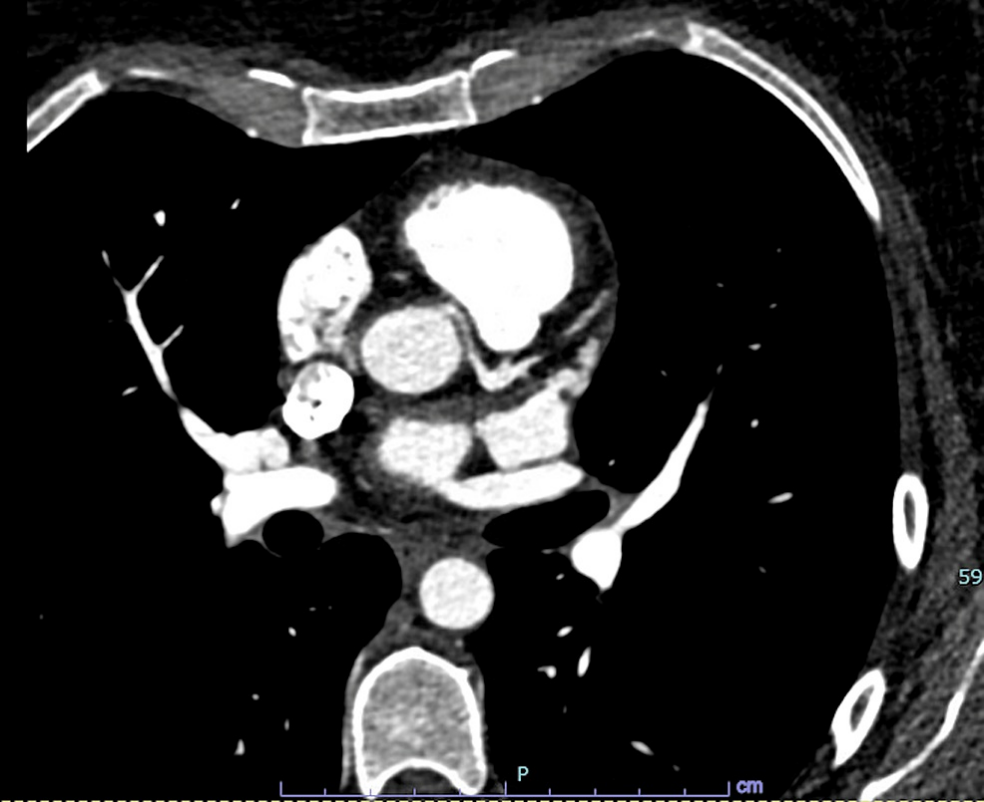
CT Angiogram Demonstrating the Interarterial Course of the Left Main Coronary Artery
